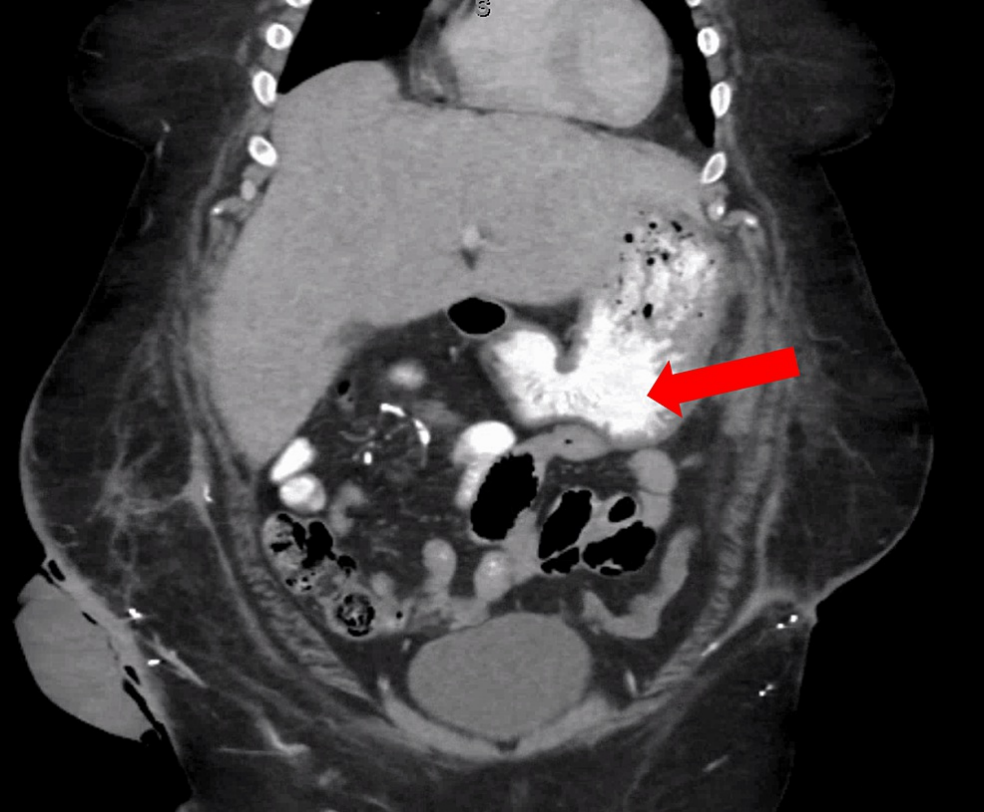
Postoperative CT scan of the abdomen with intravenous (IV) contrast showing the absence of a gastric fistula Coronal CT image of the abdomen taken two months following primary abdominal closure with the ABRA device showing enteric contrast (red arrow) in the stomach. There is no leakage of contrast into the peritoneum, indicating that the gastric fistula resolved, likely spontaneously. ABRA: Abdominal Re-Approximation Anchor
